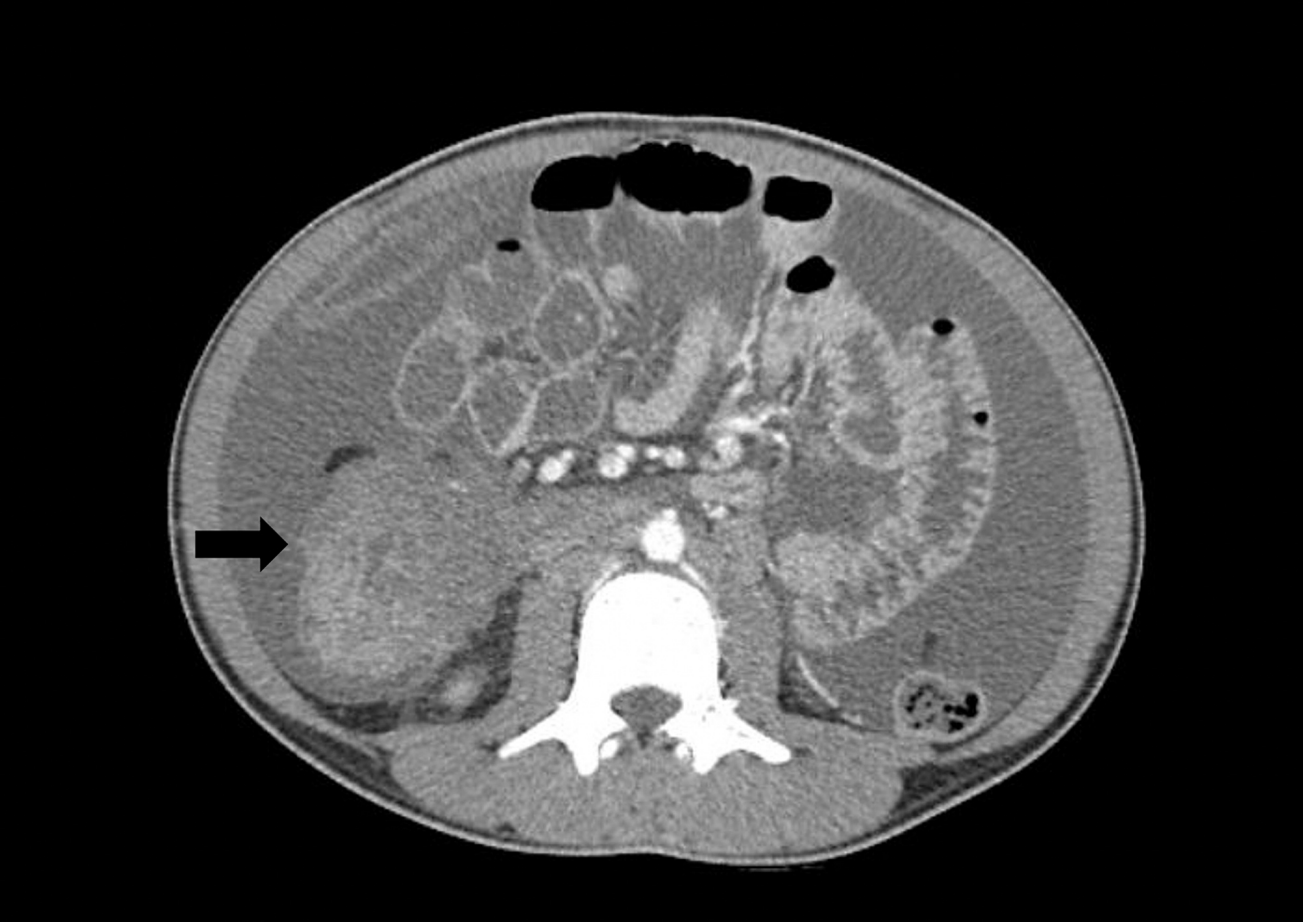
CT scan in axial view demonstrating ascites and a large ascending colon mass (arrow).
